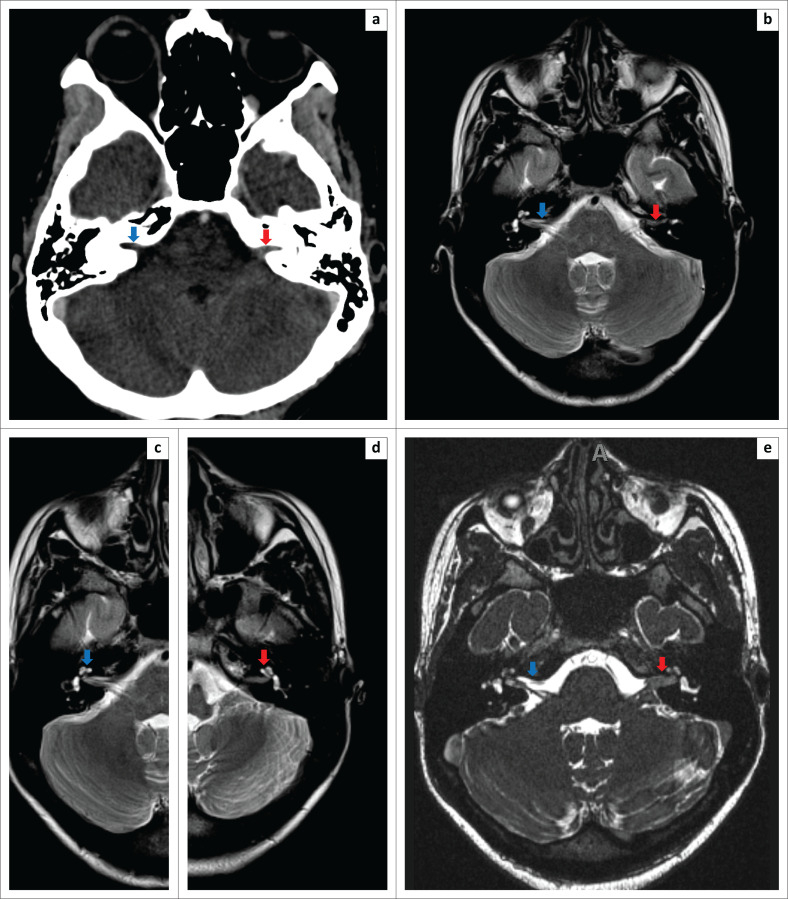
(a) Axial CT brain scan demonstrated a hyperattenuating mass at the porous acousticus of the left internal acoustic canal (red arrow). There was mild contrast enhancement and the canal was not expanded. The right internal acoustic canal appeared normal (blue arrow). (b) Axial, T2W MRI of the IAC demonstrated loss of the normal CSF signal within the left IAC (red arrow). The right IAC demonstrated signal loss to a lesser extent (blue arrow). (c–d) Axial, T2W, MRI of the cochleae demonstrated partial loss of the normal CSF signal in the basal turns of the cochleae bilaterally (blue arrow in c indicating the right and red arrow in d indicating the left cochlea). (e) Axial, MRI, constructive interference in steady state sequence, demonstrated a notable mass within the left internal acoustic canal, with loss of the normal CSF signal (red arrow). This was seen to a lesser extent in the right internal acoustic canal (blue arrow).
